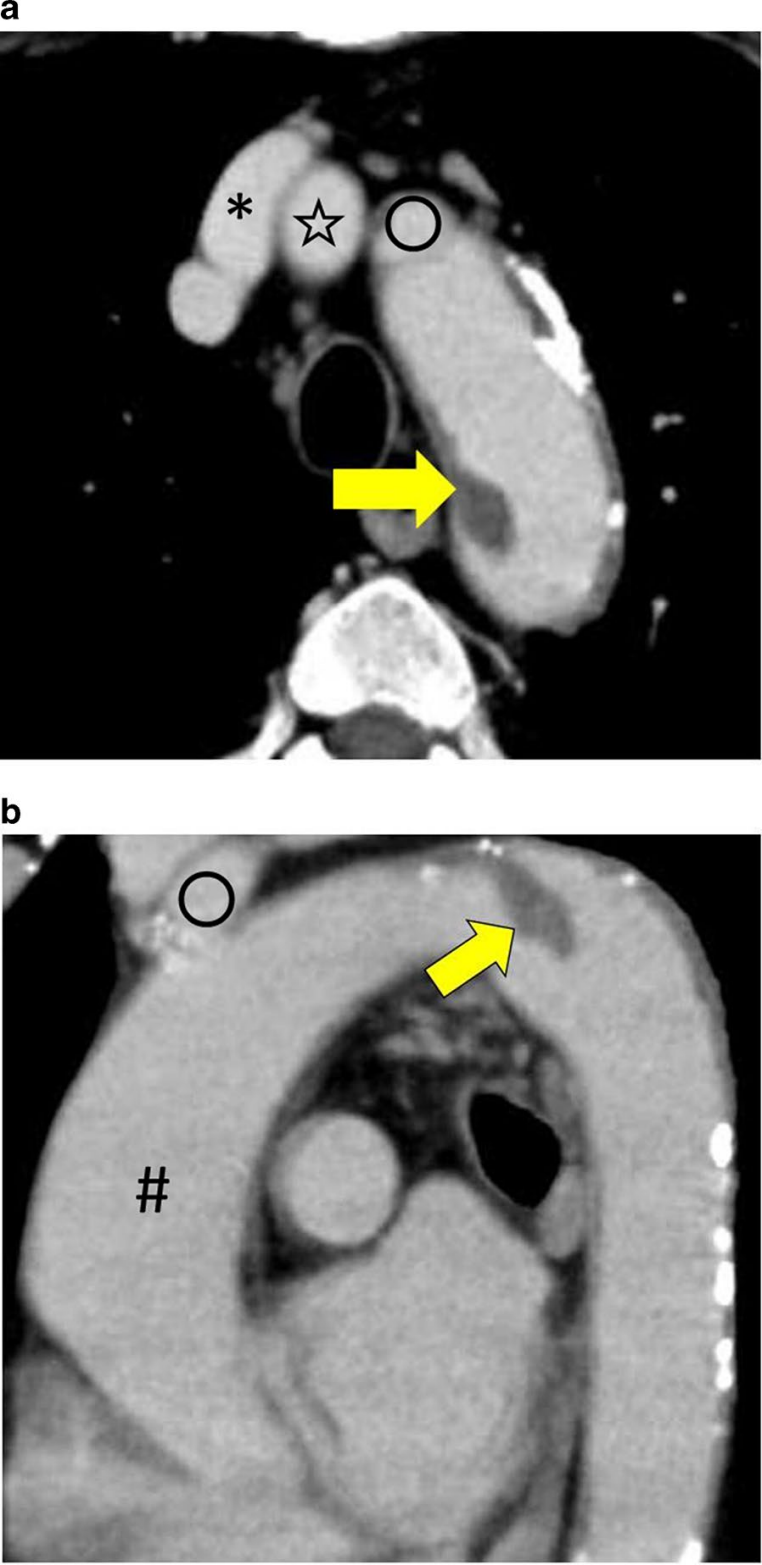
CT images. a An axial CT image showing the thrombus in the distal aortic arch (arrow). b A sagittal CT image showing the thrombus in the distal aortic arch (arrow). *Brachiocephalic artery, open star left common carotid artery, open circle left subclavian artery, # ascending aorta
